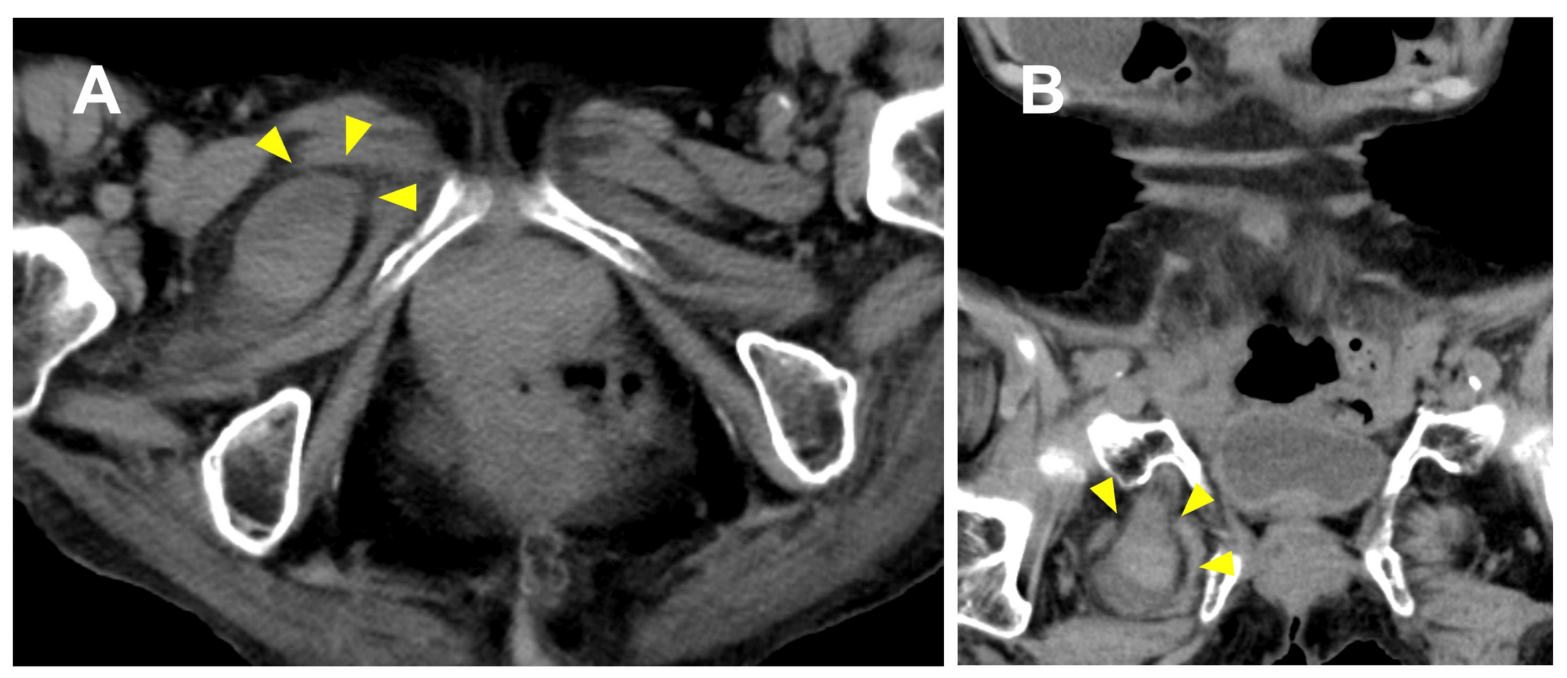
Case A: Preoperative CT. (A) Axial view showing right obturator hernia with incarcerated small bowel (arrowhead). (B) Coronal view.
